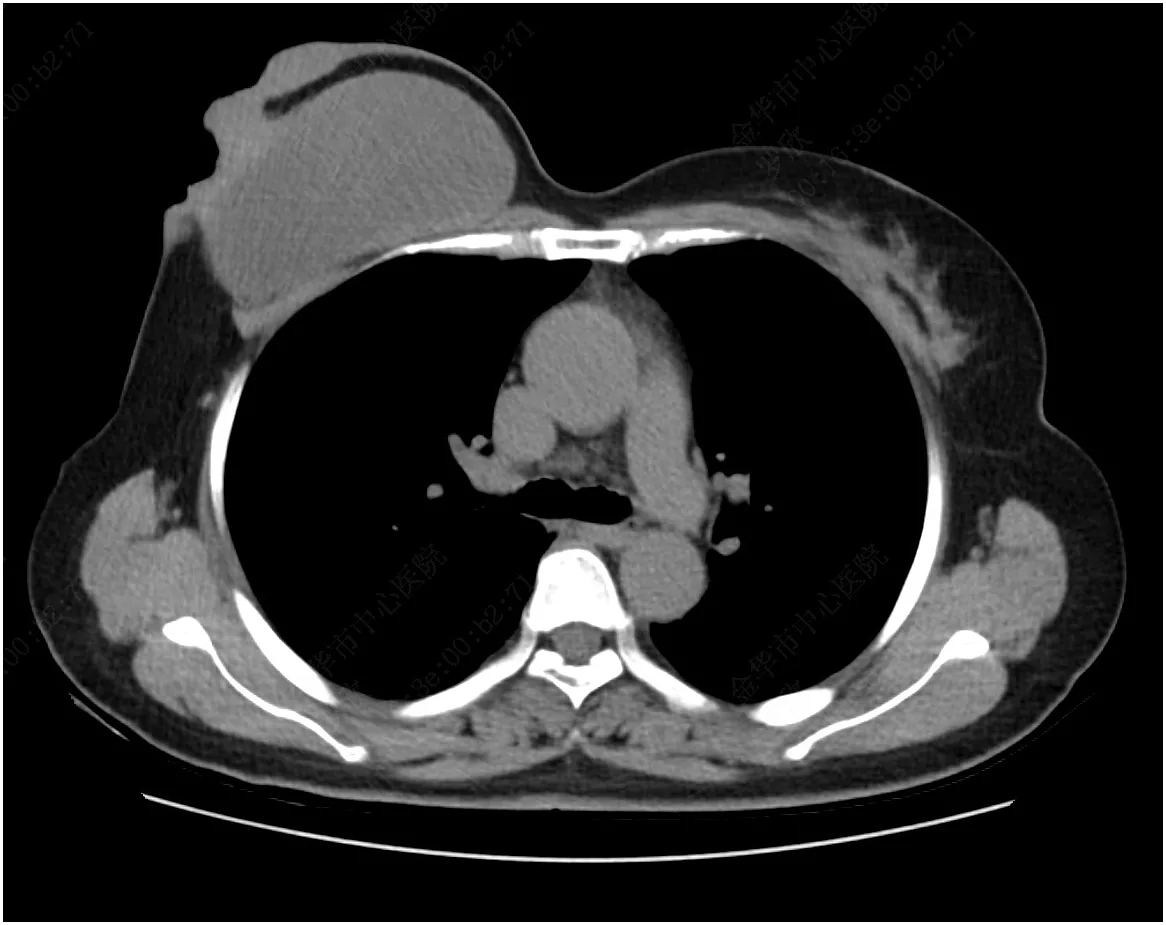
Computed tomography scan showing injected PAAG material within the right breast after augmentation, with irregular thickening of the surrounding soft tissues, multiple enlarged bilateral axillary lymph nodes, and bony destruction of the L1 vertebra.
